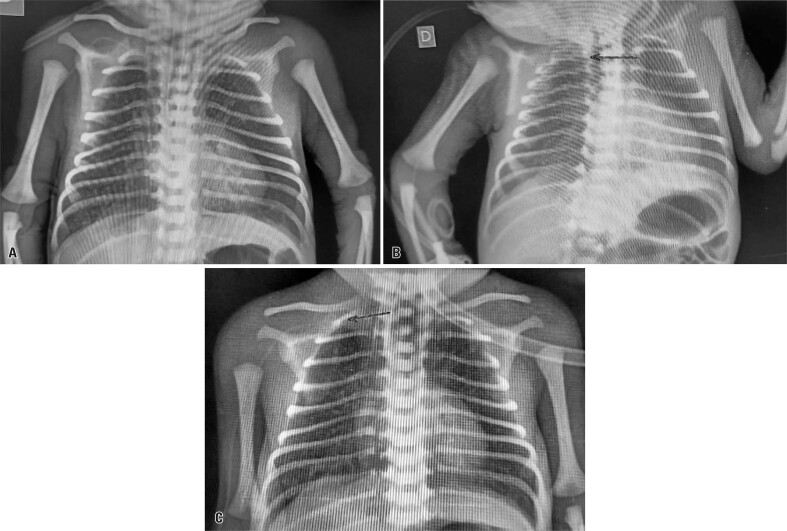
Radiographs performed after retracting the catheter by 6 cm: A) Immediately after retraction; B) 30 min after retraction; C) 24 h after retraction. The black arrow indicates the tip of the peripherally inserted central catheter
